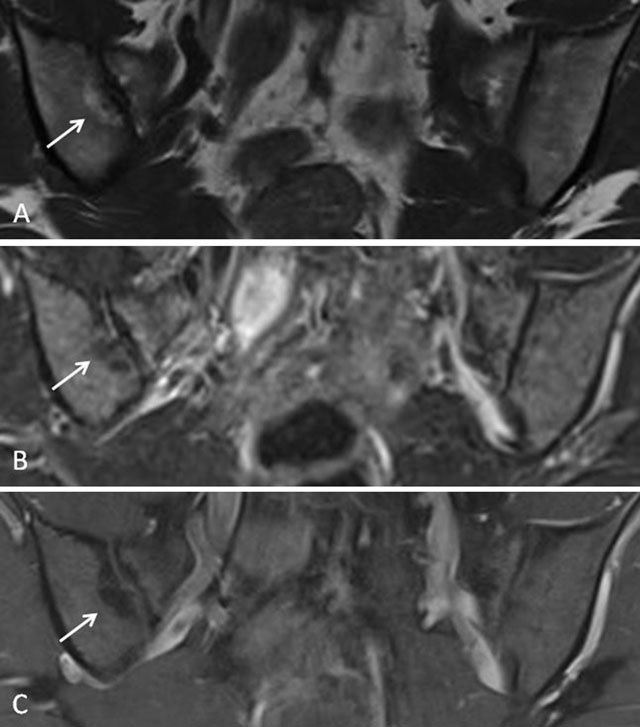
Structural lesions of sacroiliitis in a 17-year-old girl with JSpA. Semicoronal T1 (a), STIR (b), and contrast-enhanced fat-saturated T1-weighted (c) images show irregular delineation of the right SI joint with subchondral sclerosis and fat. There are also subtle erosions and subchondral sclerosis on the left side. No enhancement is seen.
